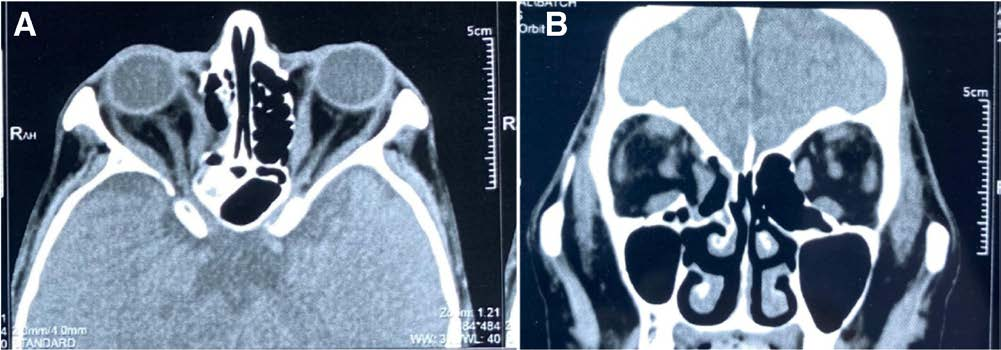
Postoperative orbital CT scan.
